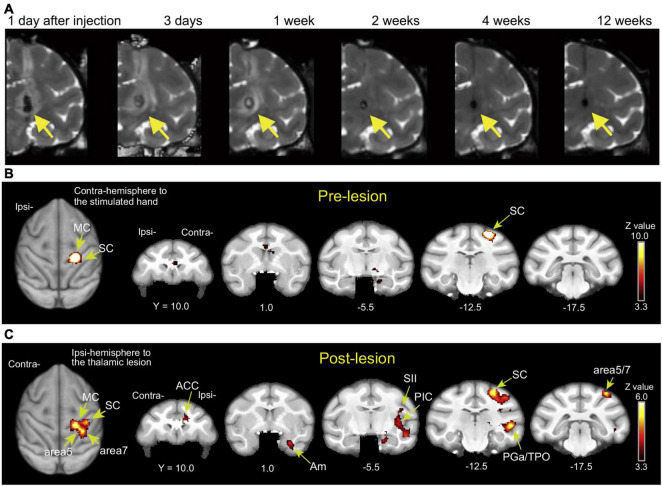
(A) T2-weighted MRI showing the time course of hemorrhagic stroke after collagenase type IV induction (coronal images). A hematoma and edema were seen as the hypointense stroke core in the VPL of the thalamus (arrows) and the surrounding hyperintense rim. Reproduced from Figure 1 of the study by Nagasaka et al. (2017). (B,C) Brain activity changes associated with CPSP. Brain activity associated with mechanical stimulation to the hand contralateral to the lesioned hemisphere (or the corresponding hand before stroke) during the pre-lesion and post-lesion periods when CPSP was developed. ACC, anterior cingulate cortex; Am, amygdala; MC, primary motor cortex; PGa, anterior subdivisions of the angular gyrus; PIC, posterior insular cortex; SC, primary somatosensory cortex; SII, secondary somatosensory cortex; TPO, temporo-parieto-occipital junction. Reproduced from Figure 3 of the study by Nagasaka et al. (2020).
